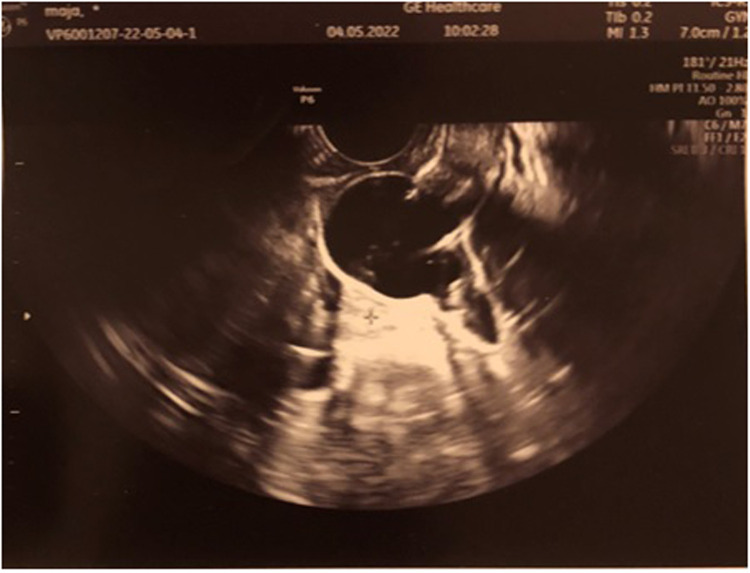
Intraoperative transrectal sonography presents hematocolpos.
